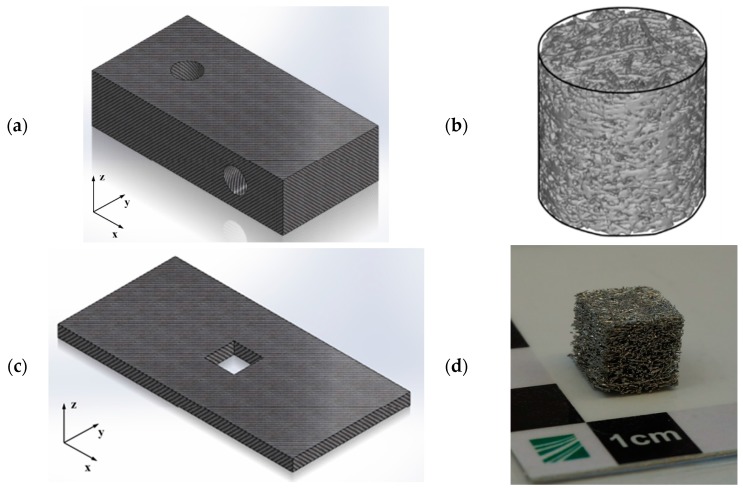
Orientation of static and dynamic test samples within the parent fiber structure: (a) brick-like parent structure used for cylindrical samples for quasi-static testing; (b) reconstructed CT image of cylindrical sample for quasi-static testing [1]; (c) plate-like parent structure for cube-shaped samples for dynamic testing; and (d) photograph of cube-shaped sample for dynamic testing. The *z*-direction corresponds to the testing direction perpendicular to the main fiber orientation.
